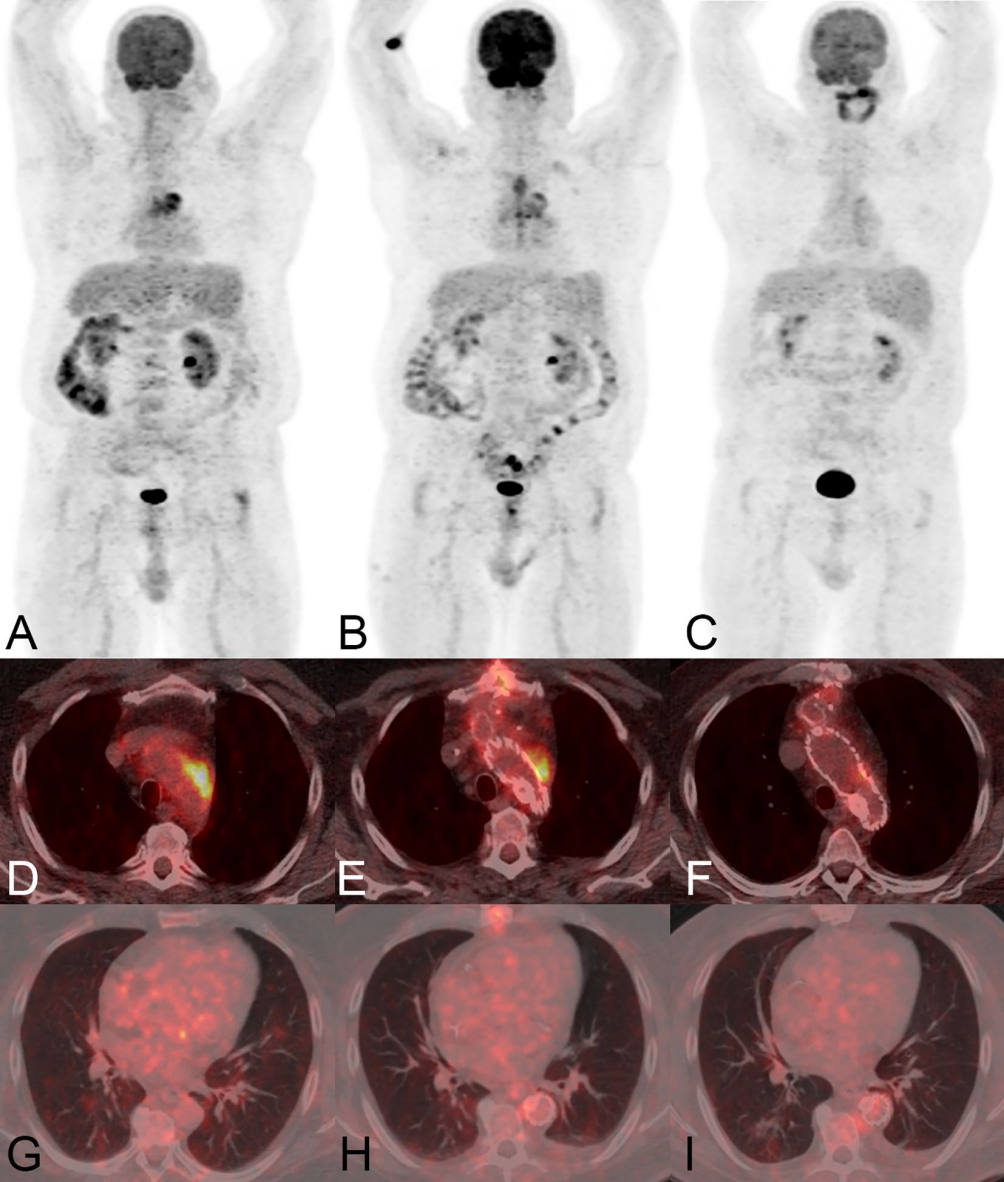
A 70-year old male patient (patient 04 in Tables 1, 2 and 3) presented with chest pain and signs for infection (C-reactive protein 122 mg/L). The initial PET/CT examination showed strongly increased FDG-uptake in the wall of an aortic arch aneurysm and no other infectious foci. Both PET/CT readers suspected a infective native aneurysm; which was clinically confirmed (*Streptococcus agalactiae* in blood cultures). At first PET/CT follow-up (ongoing antimicrobial therapy, after thoracic endovascular repair with a Conformable GORE TAG Thoracic Endoprosthesis and debranching) a strong residual focal FDG-uptake was detected adjacent to the graft, in line with a secondary vascular graft infection. The second PET/CT follow-up (ongoing antimicrobial therapy), showed only very faintly increased FDG-uptake adjacent to the graft. However, a new pneumonia in the right lower lobe was incidentally detected. The patient died 60 days after the last PET/CT due to a gastrointestinal bleeding not related to the thoracic infective native aortic aneurysm. *Note*: Panels A-C show maximum intensity reconstructions of PET; Panels D-I show fused PET/CT images. Abbreviations: PET: positron emission tomography; CT: computed tomography; FDG: 18F-fluorodeoxyglucose.
